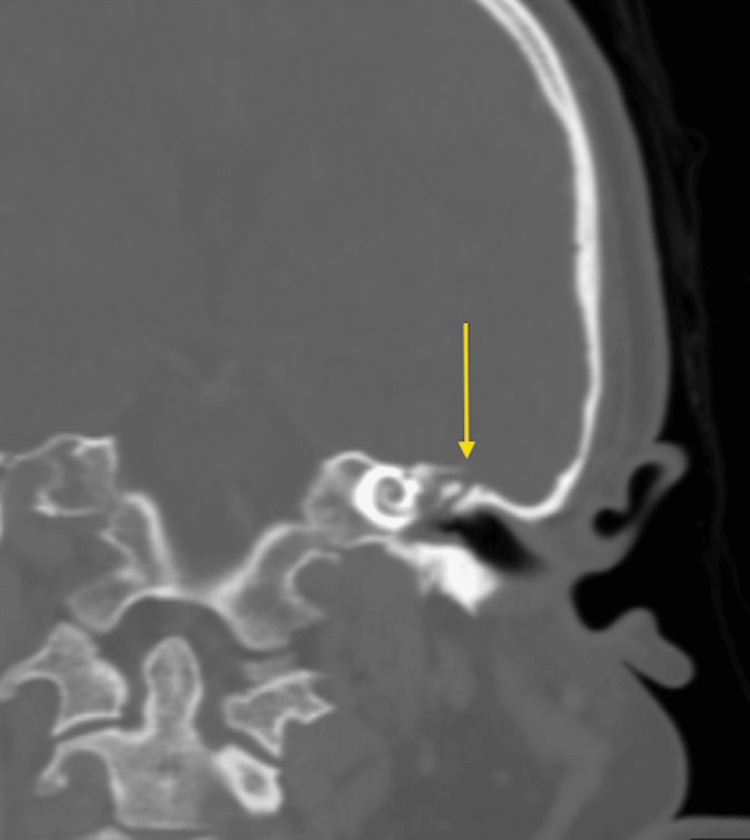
High-resolution CT Temporal Bones (coronal oblique reformat) demonstrates no definite dehiscence on the left tegmen tympani (arrow).
